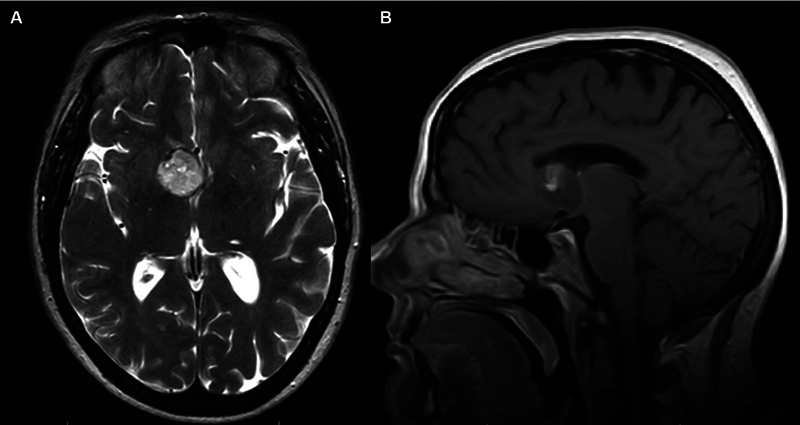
Contrast-enhanced T2-weighted axial (A) and T1-weighted sagittal (B) MRI brain suggestive of partially hemorrhagic neoplasm of the anterior septum pellucidum and right frontal lobe.
